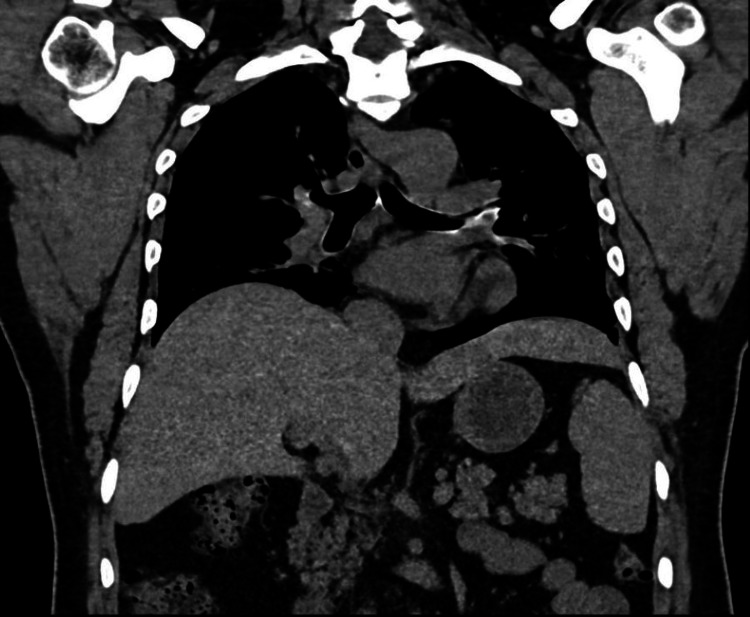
CT demonstrating beaver tail liver.
